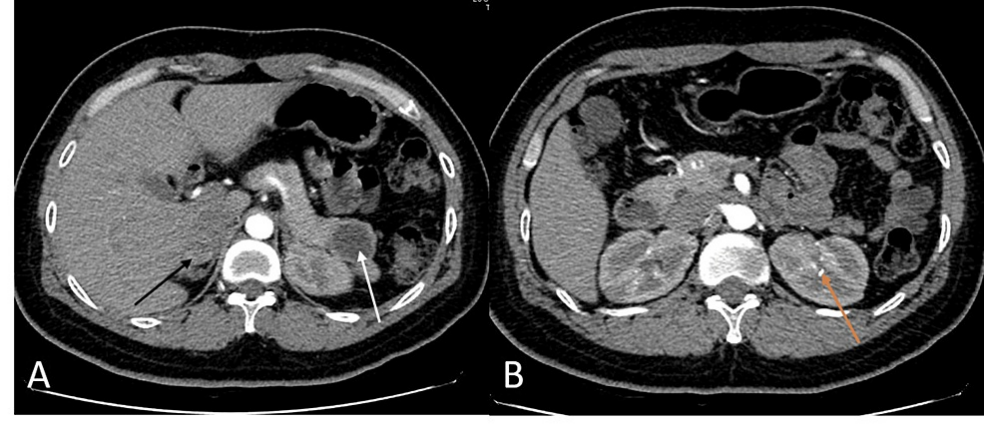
A: Axial CT showing 2.5x2.9 cm round cystic distal pancreatic mass (white arrow) and 2.0x2.7 cm oval right adrenal mass (black arrow). B: Axial CT revealing 4 mm left inter-polar renal stone (orange arrow).
